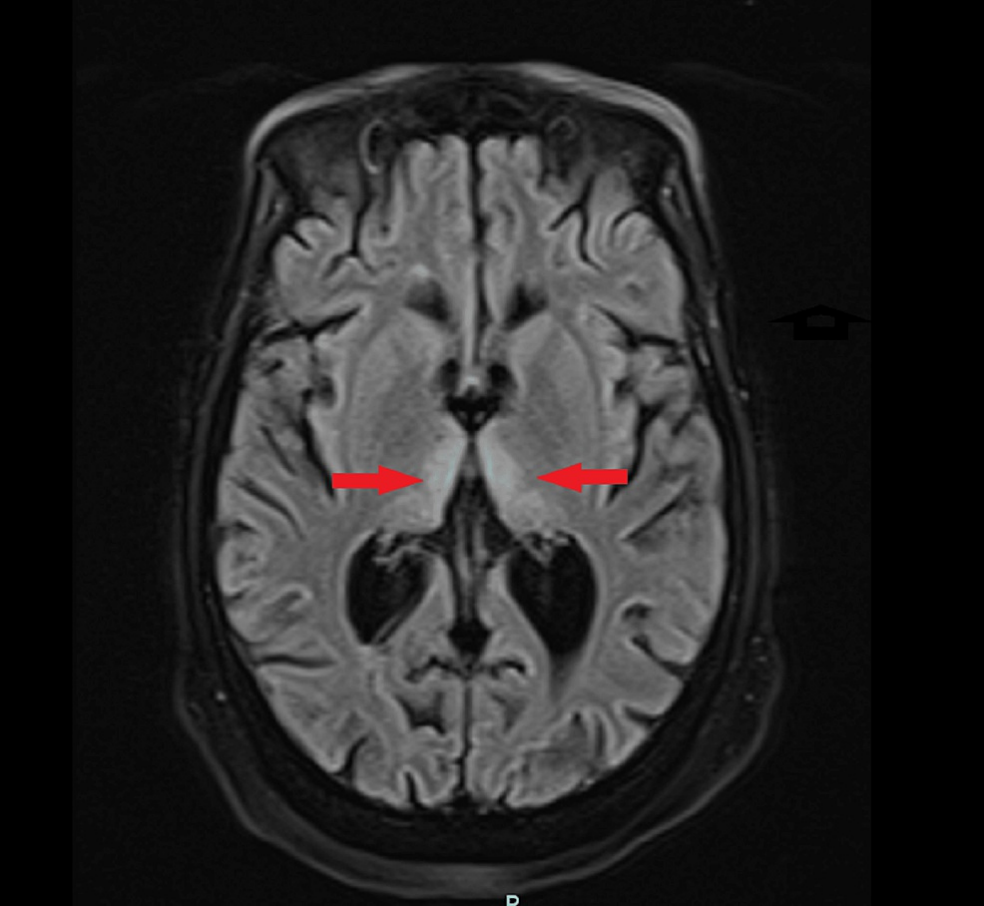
On a magnetic resonance imaging (MRI) scan of the brain, red arrows show a symmetric hypertense signal within the bilateral medial thalami region.
